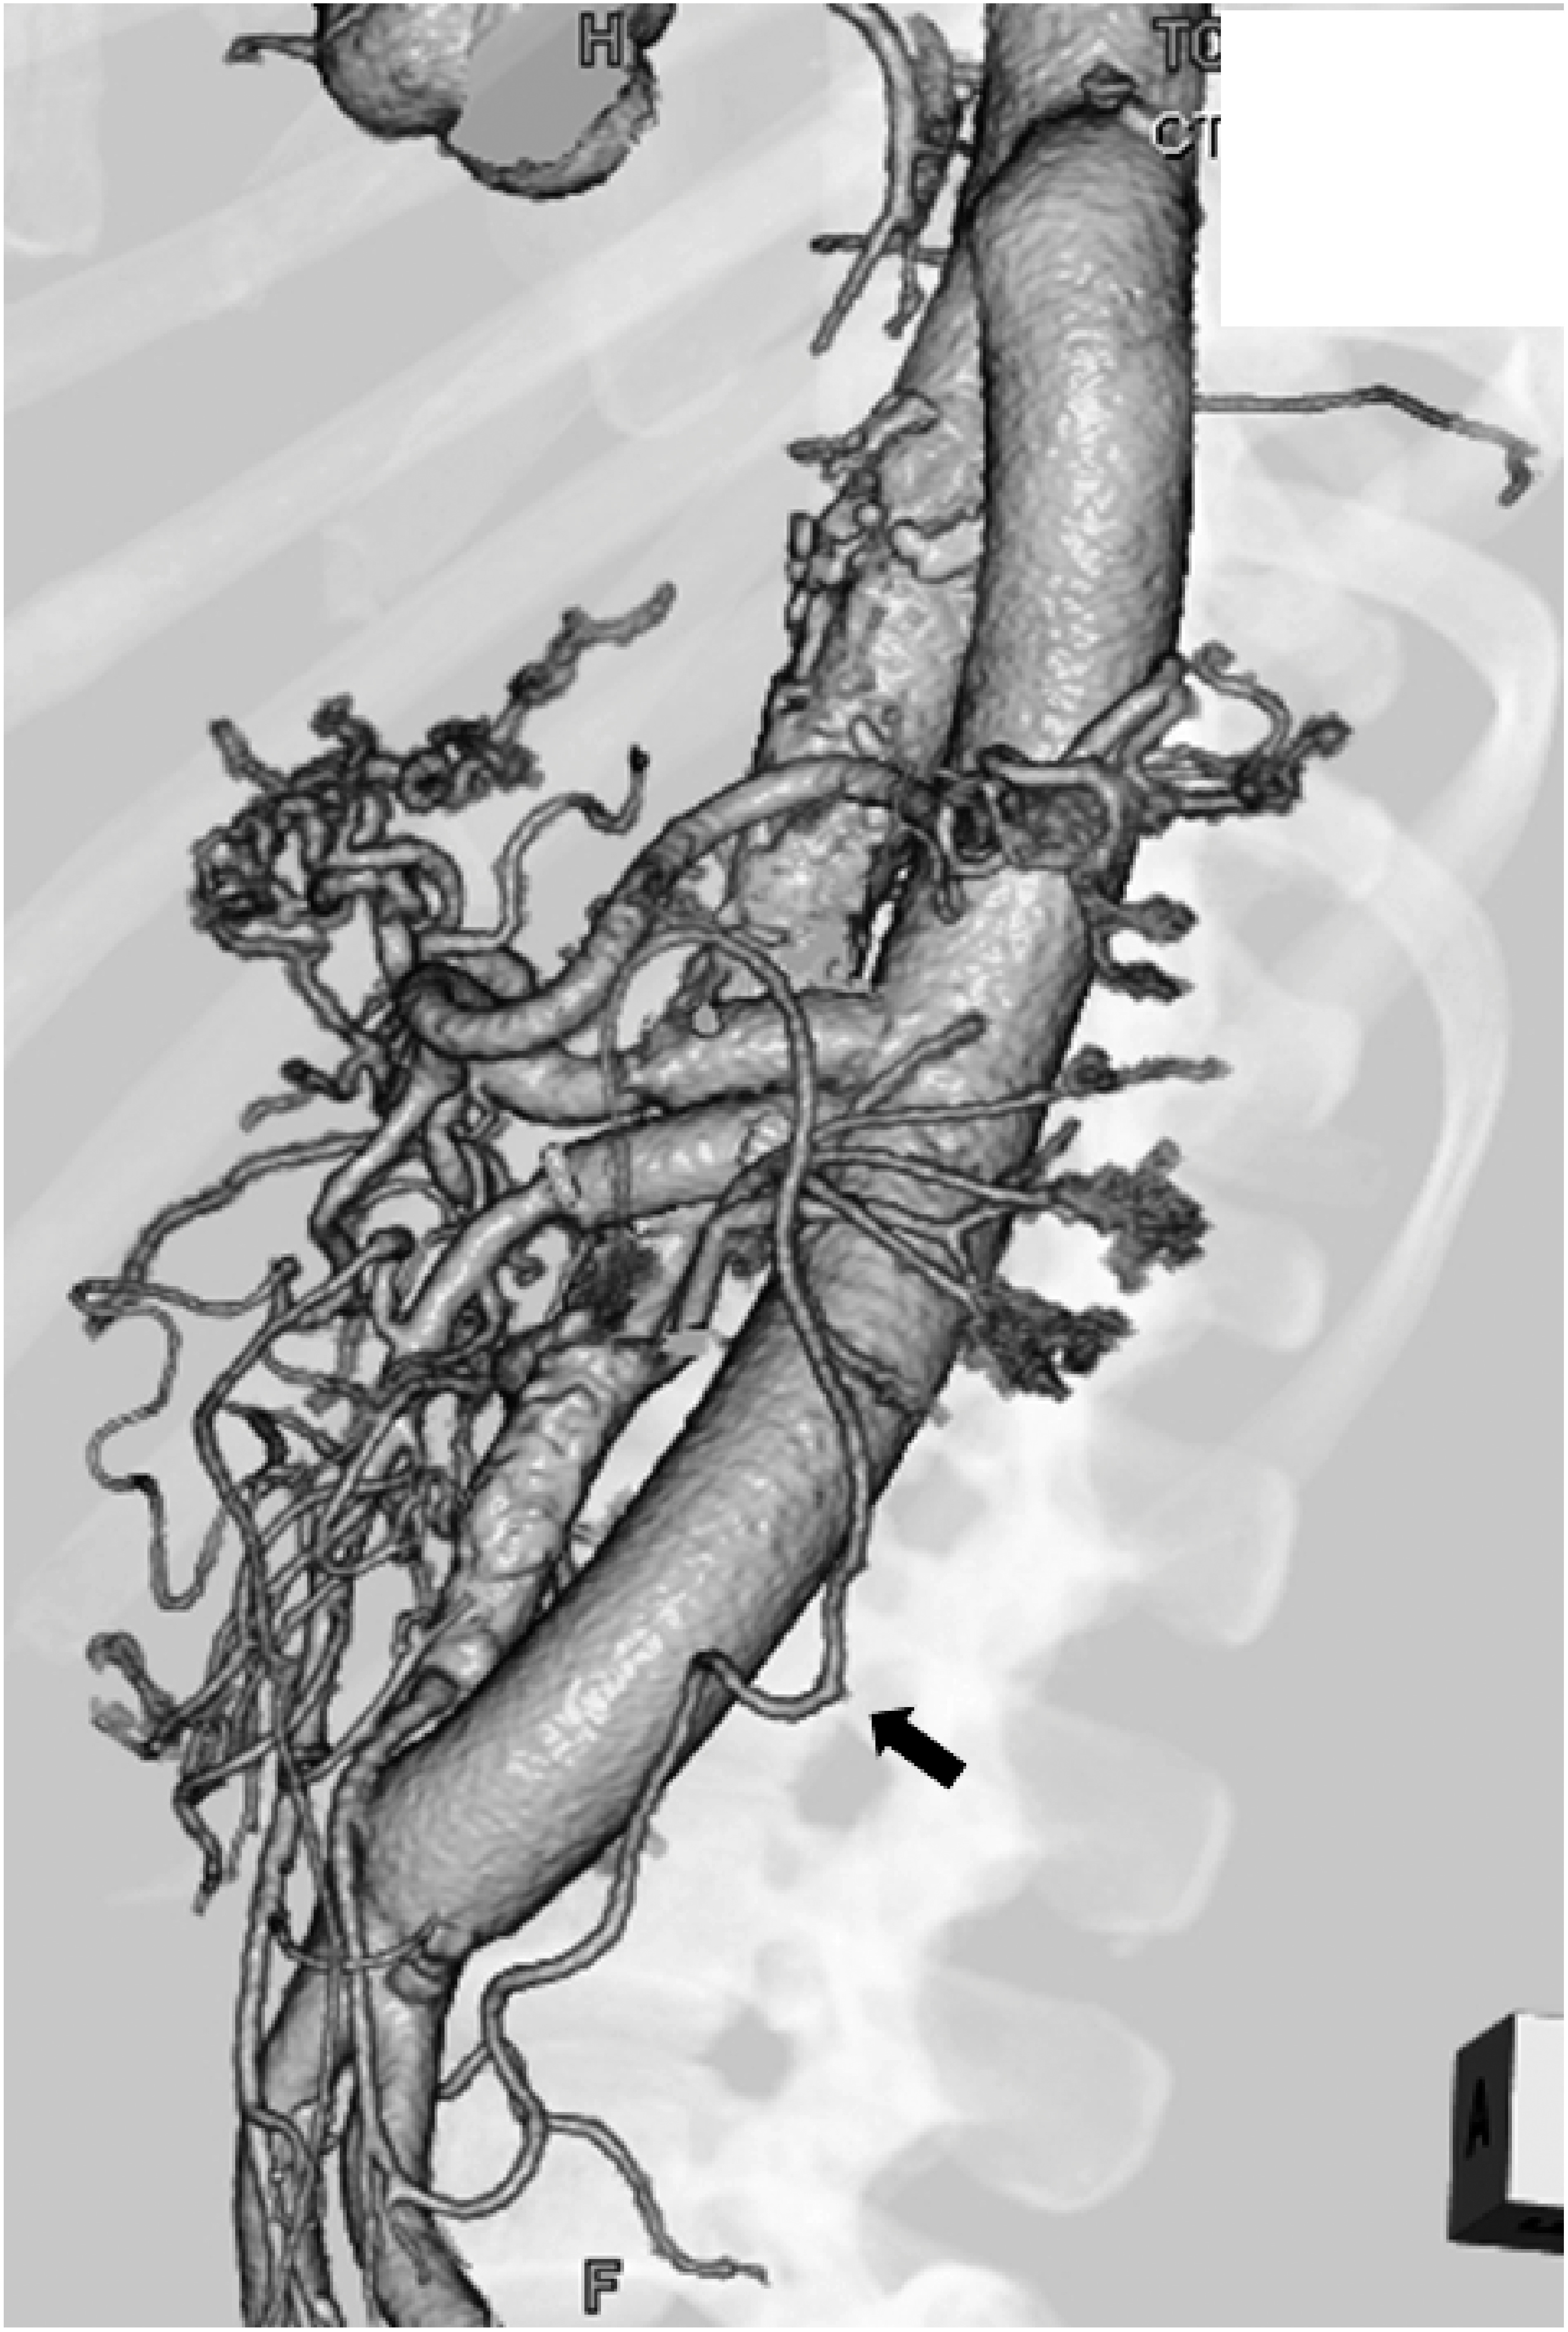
Fig. 3 Postoperative enhanced computed tomography (CT) (a volume-rendered image). No major anastomotic abnormality can be observed, and the celiac artery and superior mesenteric artery are patent. The collateral vessels from the inferior mesenteric artery seen in the preoperative CT are regressed (arrow).
